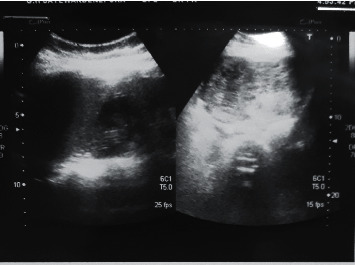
Ultrasound scan of the abdomen showing the typical snow storm appearance of the hydatidiform mole.
